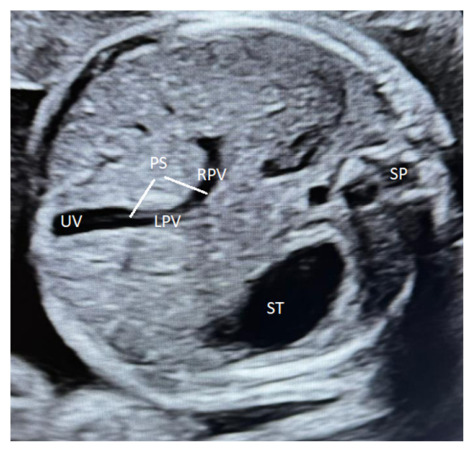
Normal intrahepatic umbilical–portal venous connection on transverse abdominal ultrasound. UV, umbilical vein; PS, portal sinus; RPV, right portal vein; LPV, left portal vein; SP, spine; ST, stomach.
